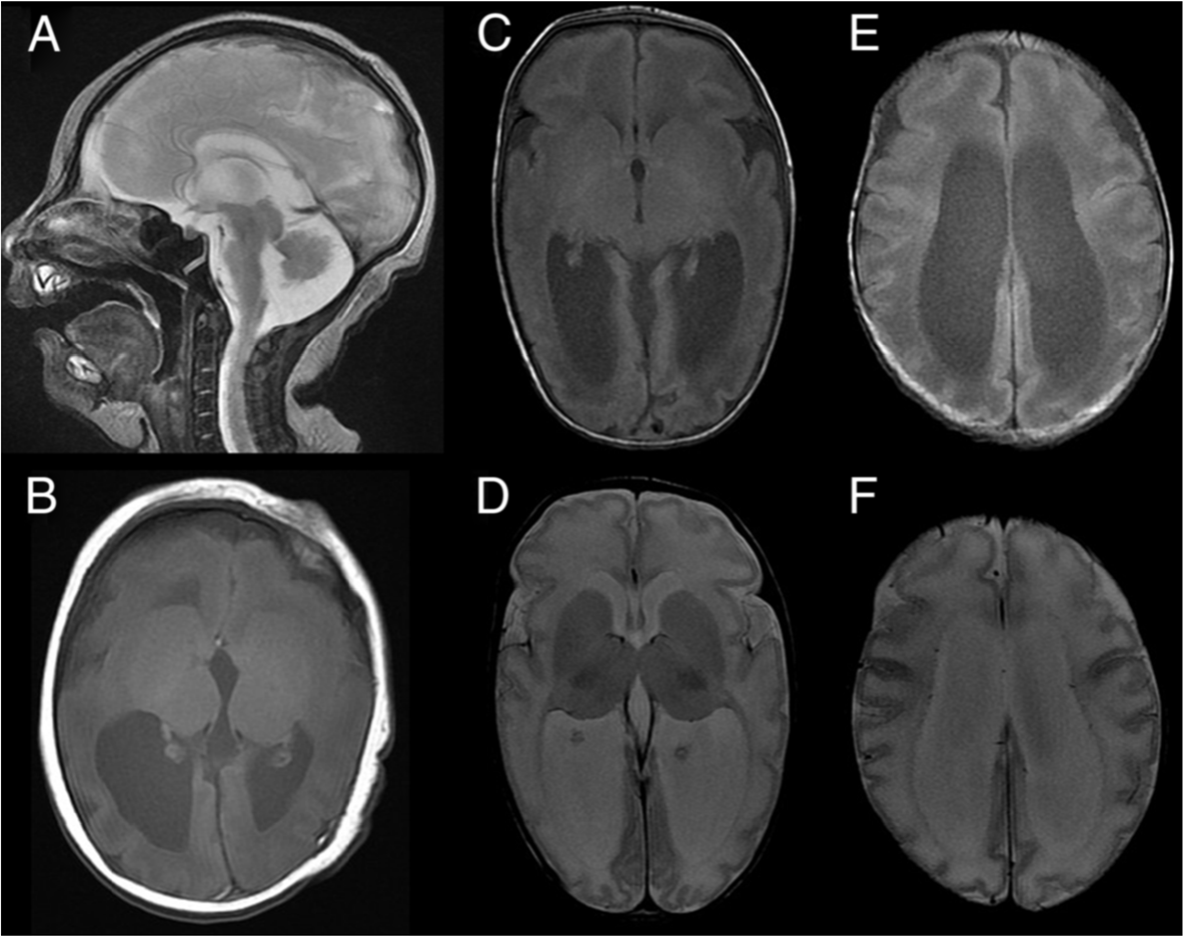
Brain magnetic resonance imaging of the patient. T2-weighted sagittal (A) and T1-weighted axial (B) images at 6 days after birth indicate hypoplastic vermis of the cerebellum, cortical dysgenesis with a simplified gyral pattern, and dilatation of the lateral ventricles. T1-weighted (C and E) and T2-weighted (D and F) axial images at 3 months of age indicate colpocephalic appearance associated with dilatation of the lateral ventricles. Cortical gyration is poor and reduced volume of the white matter is apparent.
